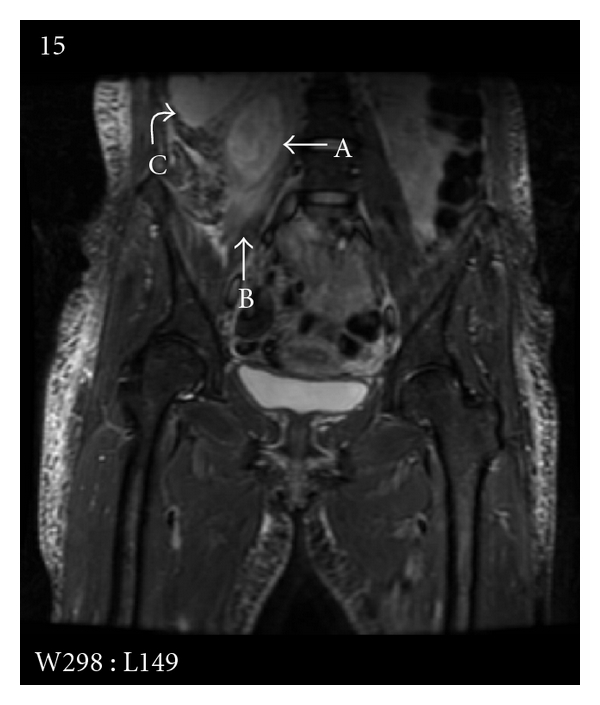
MRI, coronal view. Right-sided psoas abscess (A) with surrounding oedema (B) pushing the right kidney anterolaterally (C).
